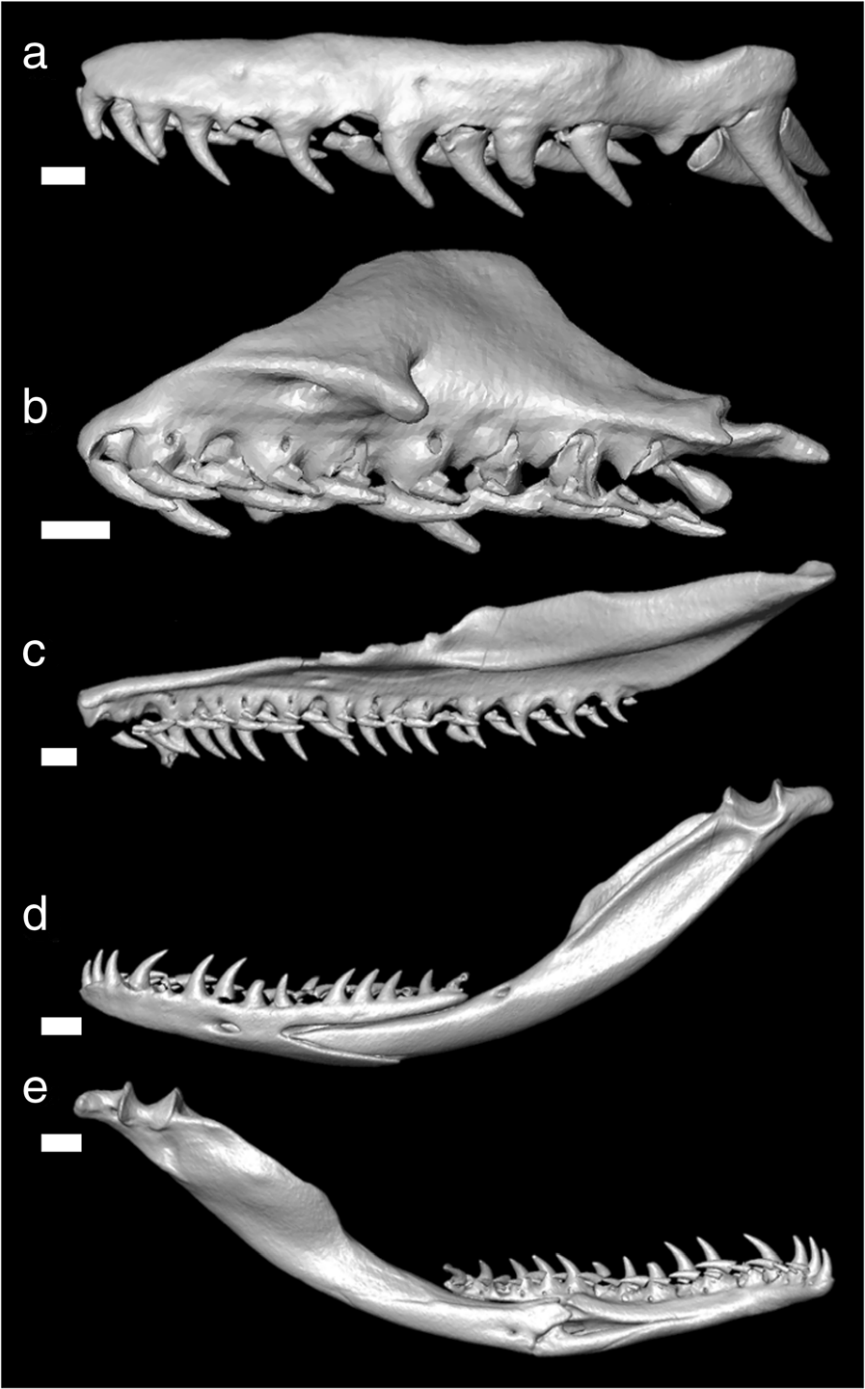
Dentition of *Leioheterodon madagascariensis* (ZSM 806/2001) based on microcomputed tomography scans of the skull. Outer lateral views of: (a) maxilla, (b) palatine, (c) pterygoid, (d) mandible (outer lateral view); (e) inner lateral view of mandible. Scale bars = 1 mm for (a–c). Scale bars = 2 mm for (d) and (e)
